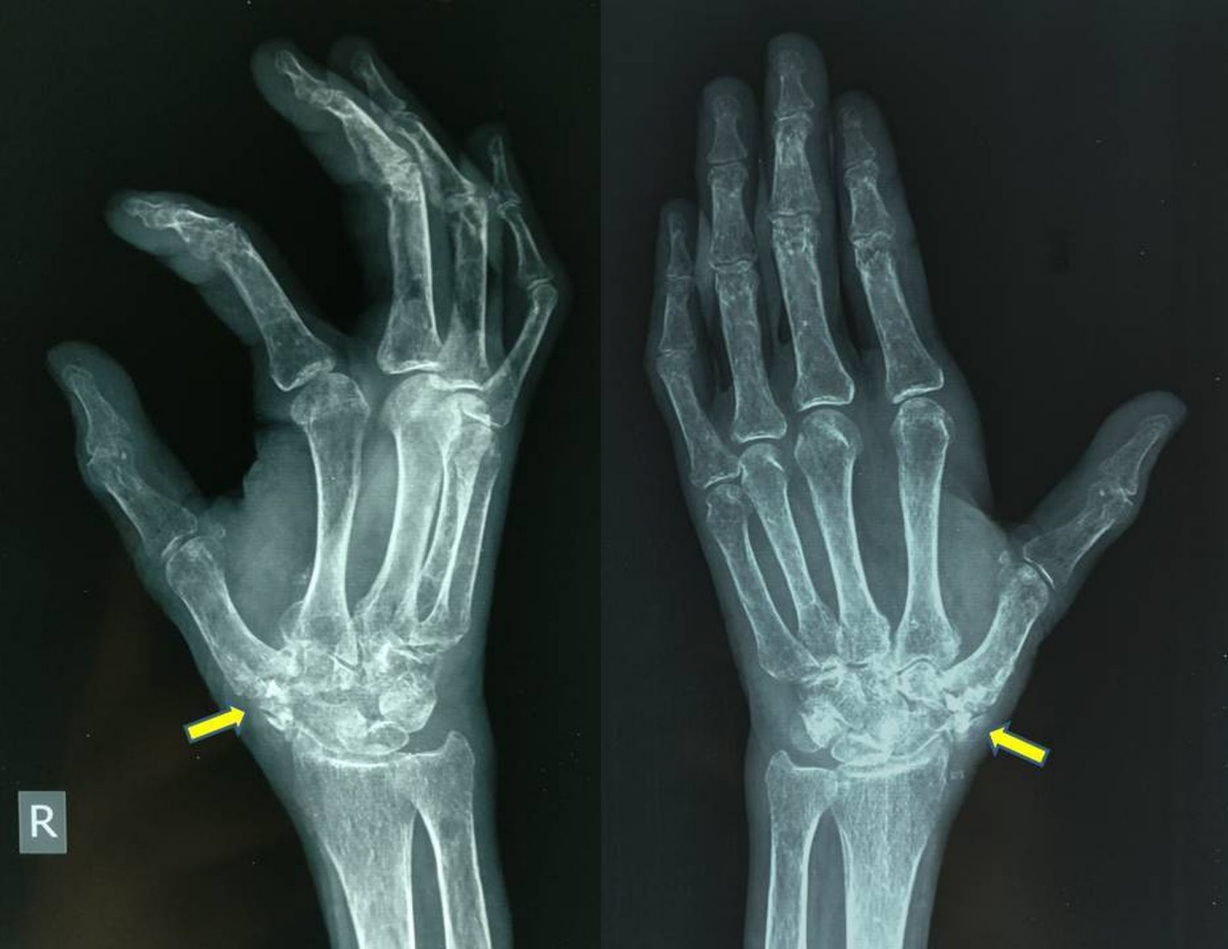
Follow-up radiograph at one year Image showing the trapezium that had gotten remodelled to create a pseudojoint.
